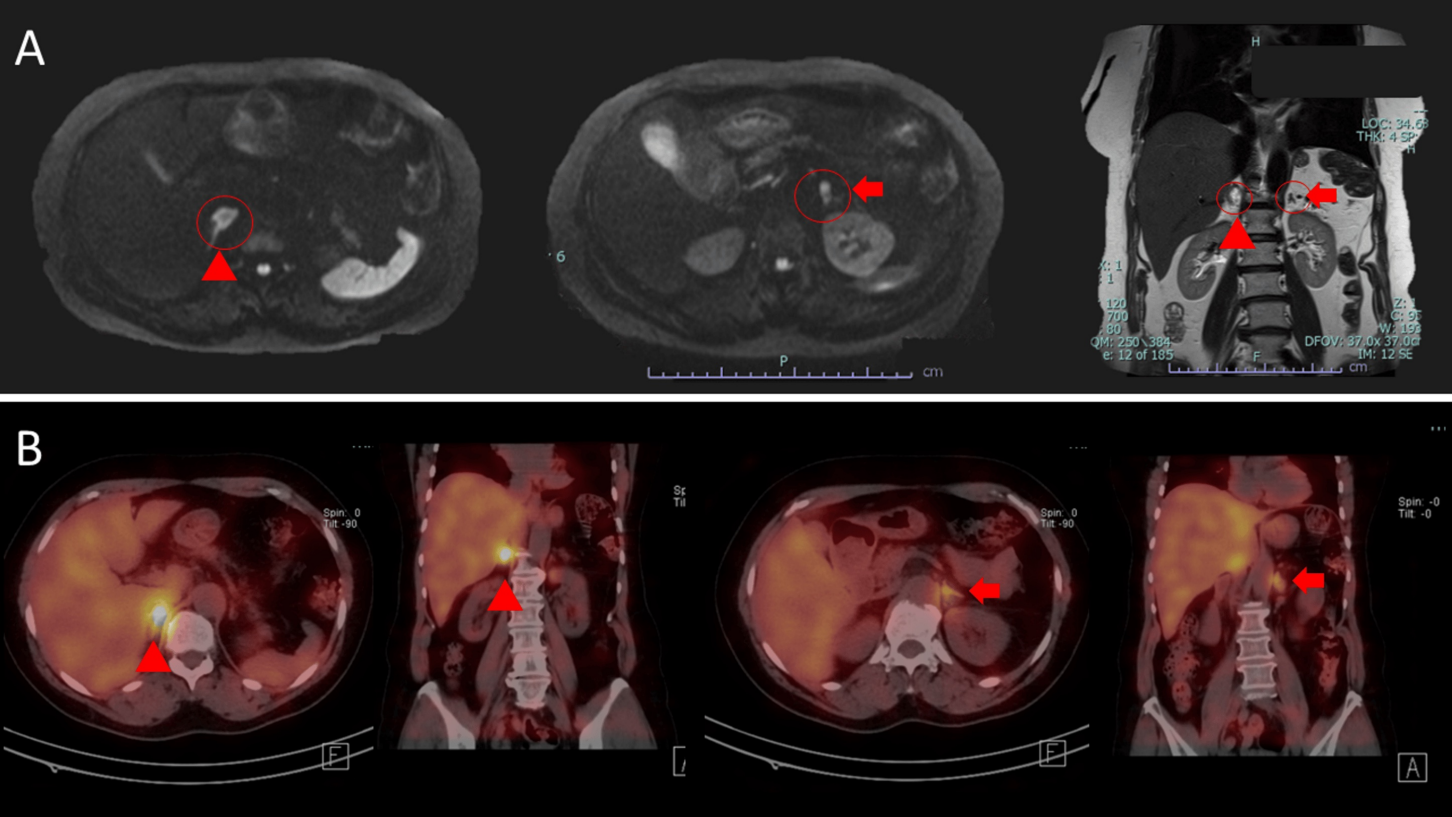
Imaging findings of the proband’s mother (A) Abdominal MRI findings showing an oval mass in front of the right kidney (▲), and a small nodule is also observed on the medial side of the upper pole of the left kidney (➡). (B) ¹³¹I-MIBG scintigraphy findings showing marked uptake in the right adrenal gland (▲), and slight uptake is also observed on the left side (➡), suggesting the possible presence of a lesion. ¹³¹I-MIBG: iodine-131 metaiodobenzylguanidine
